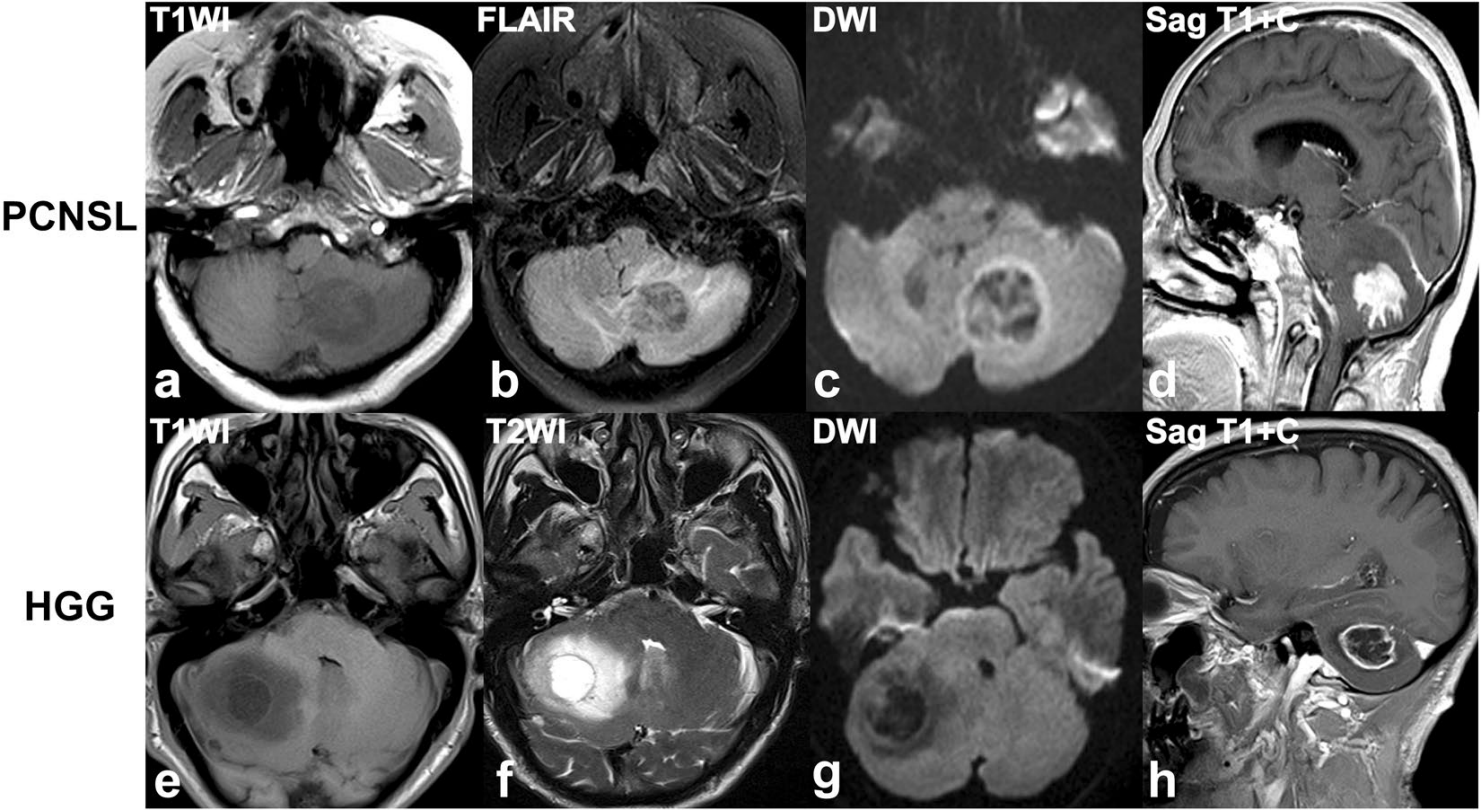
(a–d) A 58-year-old woman with a diffuse large B cell lymphoma in left cerebellum, presenting with intermittent dizziness, nausea and vomiting. (e–h). A 70-year-old man with a anaplastic astrocytoma in right cerebellum, presenting with headache, dizziness, nausea and vomiting. Axial T1W (a,e), axial fat-saturated FLAIR (b) and T2W (f), diffusion-weighted (b = 1000 sec/mm^2^) (c,g), and sagittal contrast-enhanced T1W (d,h) images reveal a homogeneously enhancing tumor in the left cerebellum (a–d), with moderate streak-like edema (b), peripheral diffusion restriction (c), and branch-like enhancement (d, arrow), and a heterogeneously enhancing tumor in the right cerebellum (e–h), with severe edema (f), hypo- in centre and iso-intensity diffusion signal in periphery of tumor (g), central cystic change and peripheral ring-like enhancement (h).
